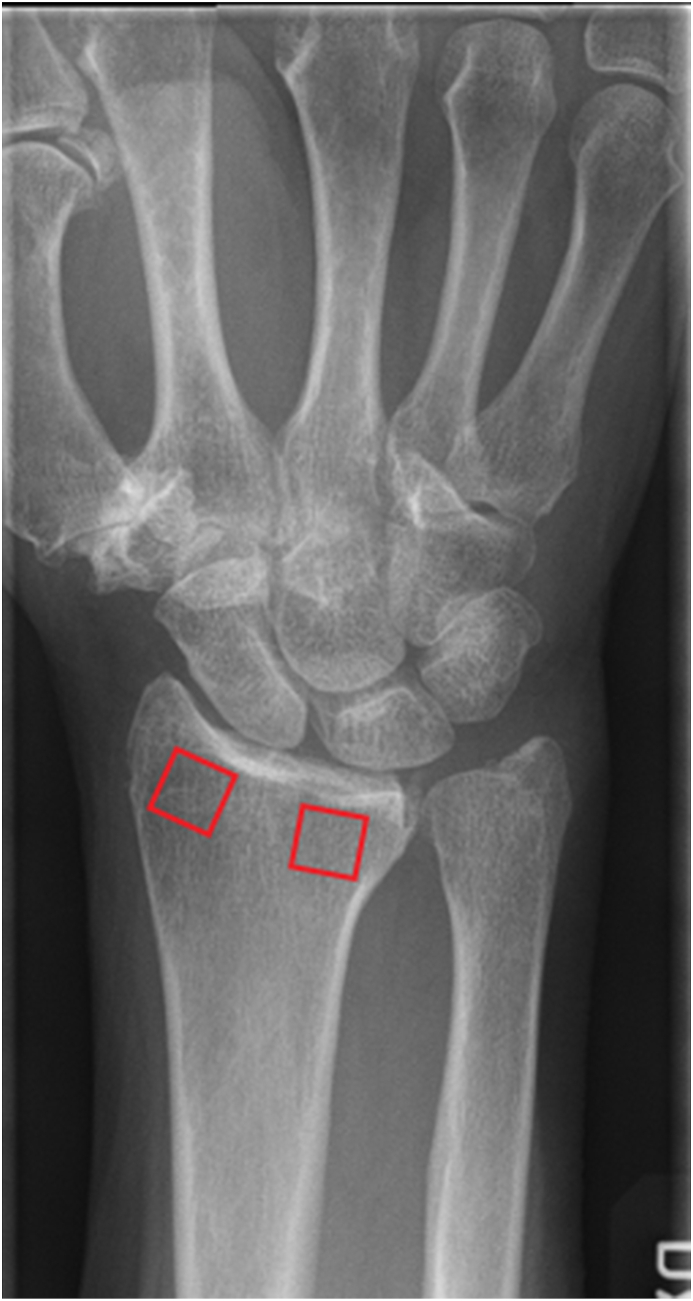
Radiograph of the wrist with the 2 selected regions of interest (ROI) marked (red squares). Visual assessment of radiocarpal osteoarthritis used the Kellgren-Lawrence classification (4 grades: 0, no osteoarthritic changes; 1, possible sclerosis and minimal osteophytes without joint space narrowing; 2, slight joint space narrowing and osteophytes; 3, moderate joint space narrowing, osteophytes, and possible cyst formation; 4, severe joint space narrowing, osteophytes, and sclerosis).
